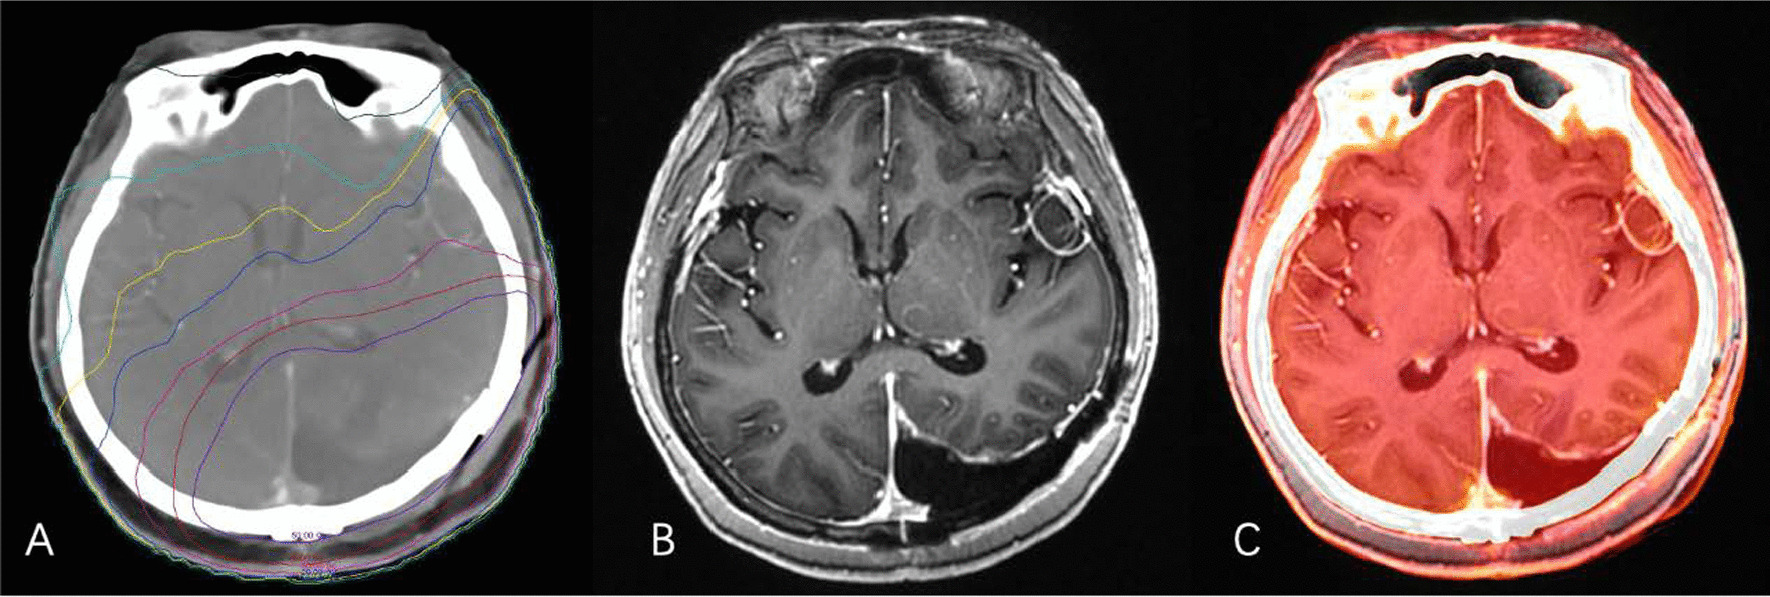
Registration of image fusion. A: Radiation dose distribution on a CT image; B: MR T1+C image; C: Fusion of the registered images
